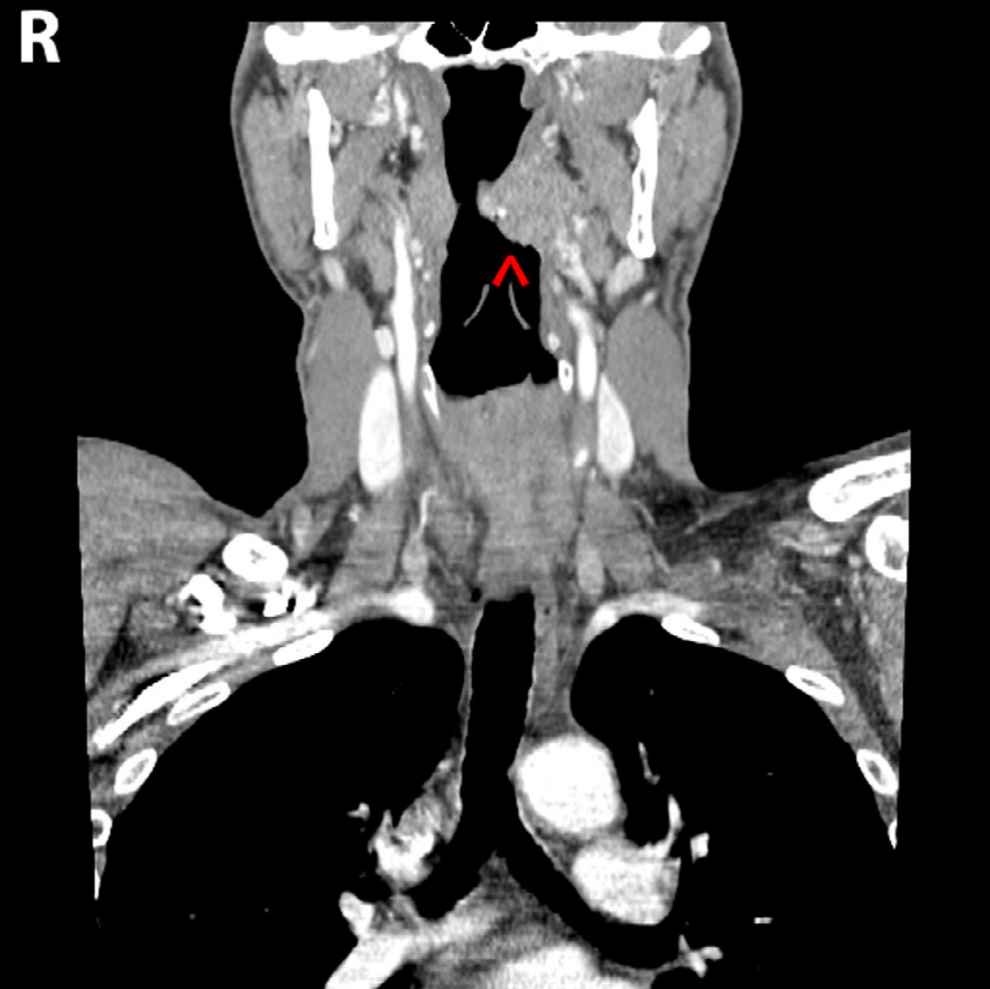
Computed tomographic (CT) neck with contrast. Coronal view showing the exophytic mass (red arrow) extending medially towards the uvula within the oropharynx
